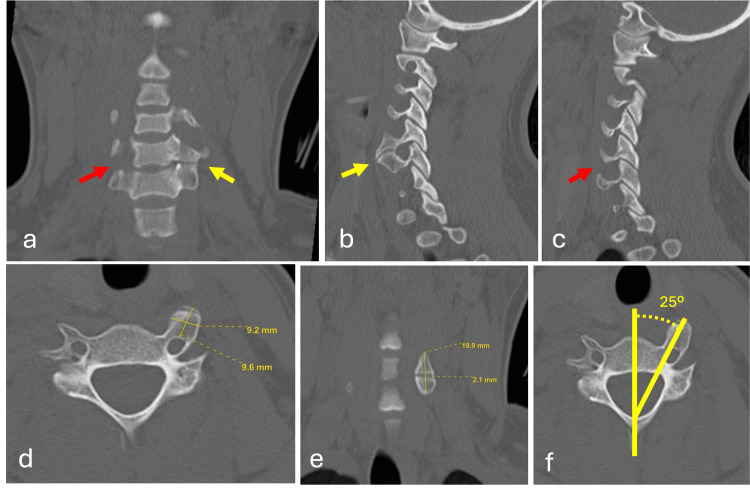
Multiplanar CT images demonstrating accessory articulation of elongated anterior tubercles of the left transverse processes at C5-C6. (a) Coronal reformatted CT image demonstrates asymmetric elongation of the anterior tubercles of the left C5 and C6 transverse processes forming a well-corticated accessory articulation (yellow arrow). The normal right-sided anterior tubercles of the C5 and C6 transverse processes are indicated for comparison (red arrow). No acute fracture or subluxation is identified. (b) Left parasagittal reformatted CT image shows the elongated anterior tubercles of the C5 and C6 transverse processes with a smooth, well-corticated accessory articulation between them (yellow arrow). (c) The right parasagittal reformatted CT image demonstrates normal morphology of the right C5 and C6 anterior tubercles of the transverse processes (red arrow), providing contralateral comparison to the left-sided variant. (d) Axial CT image at the C5–C6 level demonstrates the elongated left anterior tubercle of the transverse process projecting anterolaterally. Annotated measurements show the elongated anterior tubercle length (9.2 mm) and the total anteroposterior transverse process dimension (9.6 mm). The left transverse foramen is patent. Note the normal morphology of the right transverse process for comparison. (e) Coronal reformatted CT image with annotated measurements demonstrates the craniocaudal length of the combined elongated anterior tubercles and accessory articulation (19.9 mm) and the joint space width (2.1 mm) at the articulation. (f) Axial CT image with annotated measurement demonstrates the angle of projection of the elongated left anterior tubercle relative to the sagittal vertebral body axis (25°). The anterolateral projection is directed away from the expected course of the cervical nerve roots and vertebral artery within the transverse foramen.
